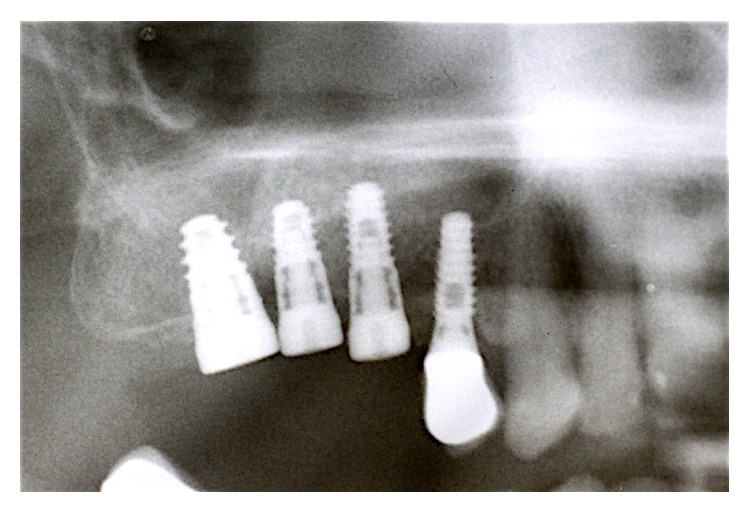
Radiographic evaluation after implant placement.
